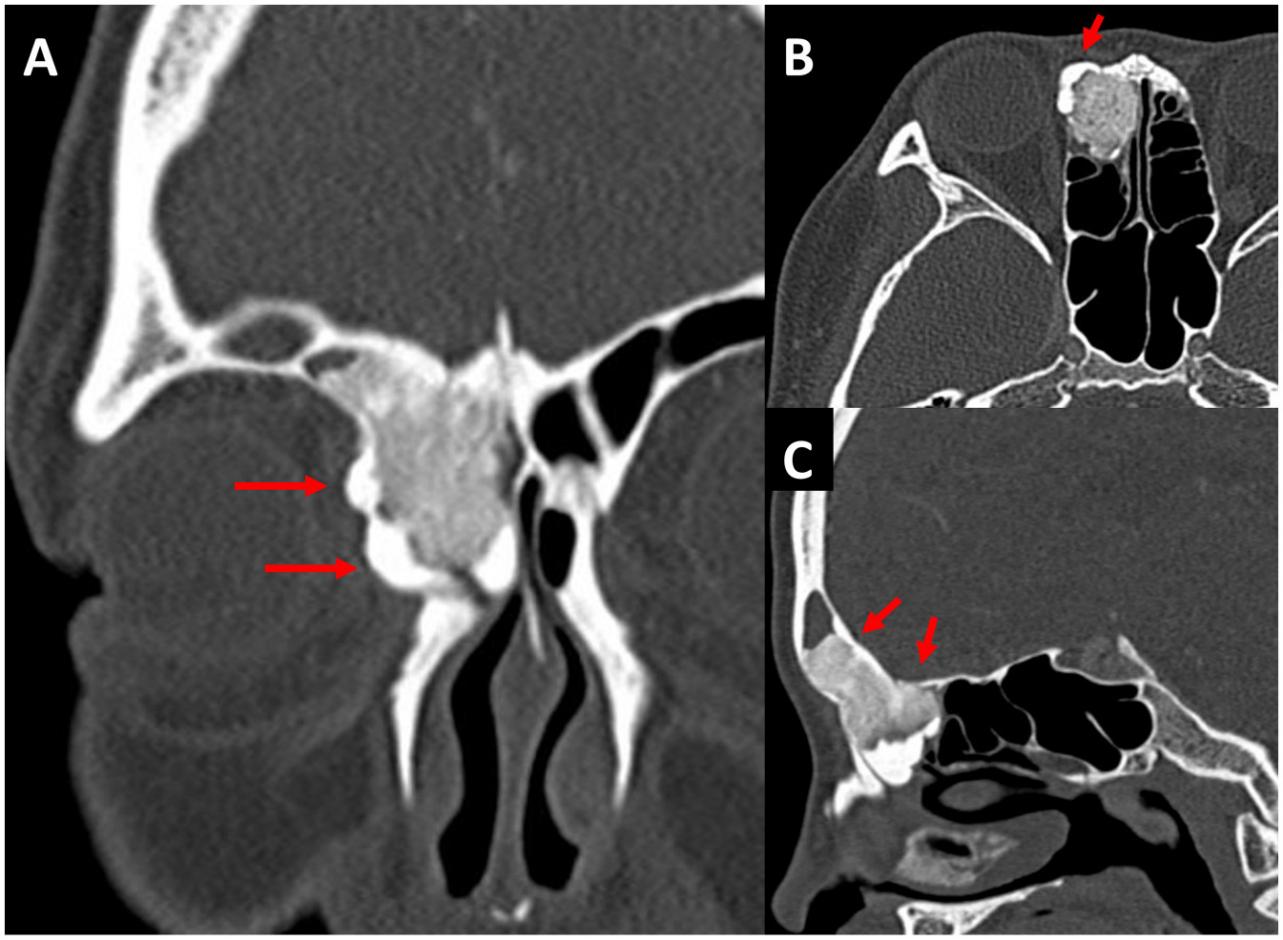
Coronal (A) computed tomography (CT) demonstrates large right sinonasal osteoma with apparent deformity of the right medial orbital wall (arrows) with mass effect on orbital structures. Axial slice (B) noting area of palpable bony protuberance to medial orbit palpated on examination (arrow). Sagittal cut through right paranasal sinuses demonstrating near complete filling of frontal sinus and anterior ethmoid involvement (C) by osteoma with broadly based skull base attachment (arrows).
